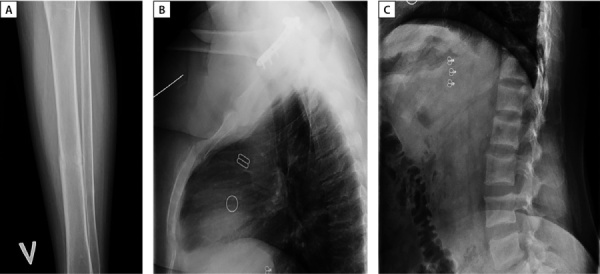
Рисунок 1. А — рентгенография левой голени в прямой проекции. Консолидированный перелом в средней трети без смещения. В — рентгенография грудного отдела позвоночника в боковой проекции. Гиперкифоз, умеренный остеохондроз, спондилез. Начальная компрессия тел Th7, Th8 позвонков по краниальной и каудальной площадкам преимущественно в передней трети позвонков (потеря до 5% массы). С — рентгенография поясничного отдела позвоночника в боковой проекции. Гиперлордоз, остеохондроз, ретролистез L4 и L1 на 2 мм. Начальная компрессия тел L5 (до 8% потери массы по каудальной площадке в задней трети позвонка), L3 ( до 5% в средней трети), L2 ( до 8% в средней трети) позвонков.Figure 1. A — X-ray of the left leg in direct projection. Consolidated fracture in the middle third without displacement. C — X-ray of the thoracic spine in lateral projection. Hyperkyphosis, moderate osteochondrosis, spondylosis. Initial compression of the bodies of Th7, Th8 vertebrae along the cranial and caudal sites, mainly in the anterior third of the vertebrae (loss of up to 5% of the mass). C — radiography of the lumbar spine in lateral projection. Hyperlordosis, osteochondrosis, L4 and L1 retrolisthesis by 2 mm. Initial compression of the bodies of L5 (up to 8% weight loss along the caudal area in the posterior third of the vertebra), L3 (up to 5% in the middle third), L2 (up to 8% in the middle third) of the vertebrae.
